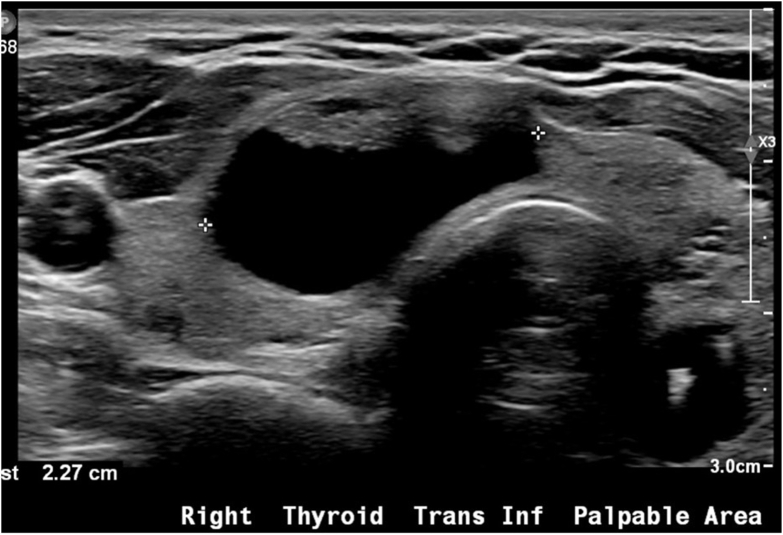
Thyroid ultrasound image of *right* thyroid cystic nodule. The nodule measured 2.3 × 1.3 × 2.3 cm and was TI-RADS 2. It had no internal vascularity.
